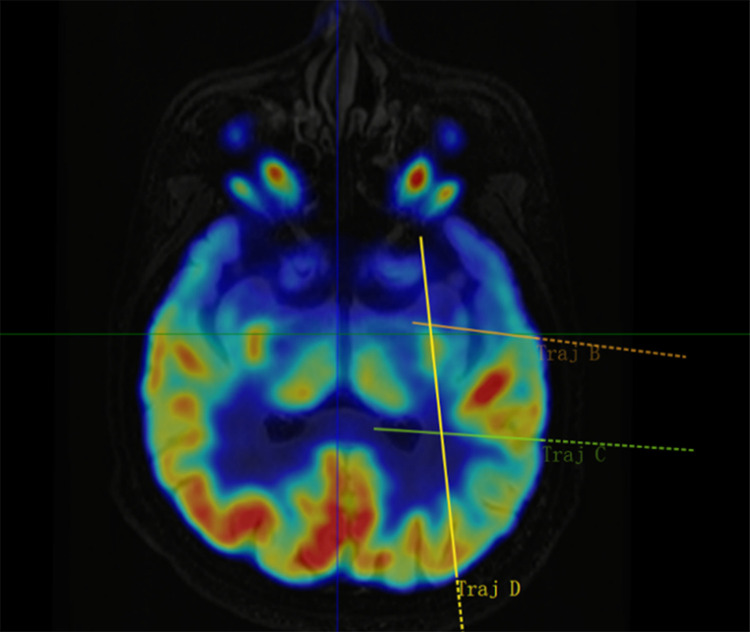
Co-registered axial MRI T2 FLAIR and PET-CT images.
